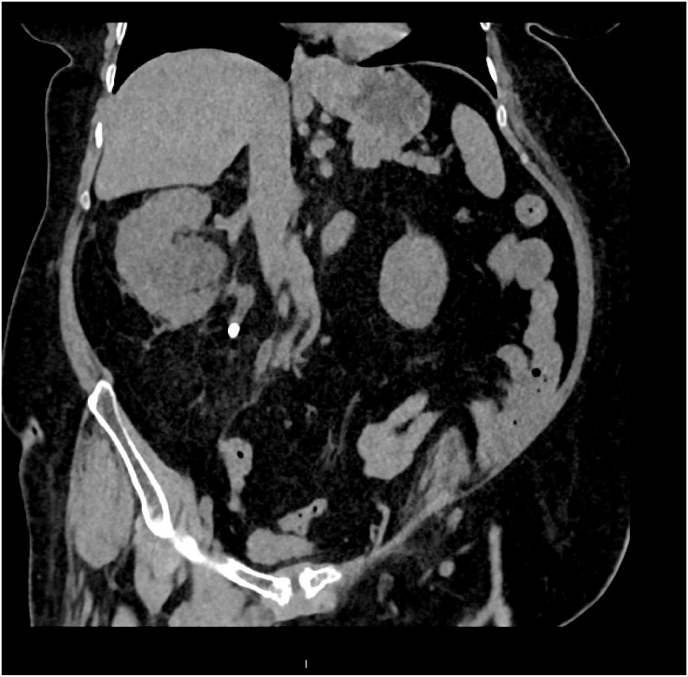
Coronal non-contrast CT scan showing 10 mm obstructive Right proximal ureteric calculus and gross right hydronephrosis.
